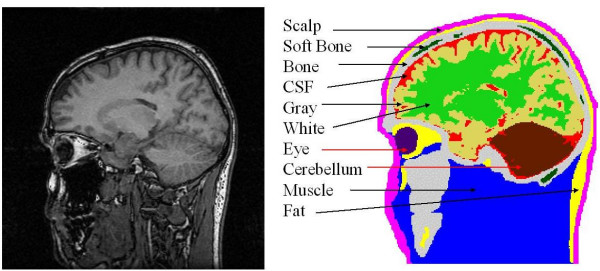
(Left) A raw MRI slice, (right) segmented slice with ten major tissues identified in it. The soft tissue which is present in other slices is not included here.
